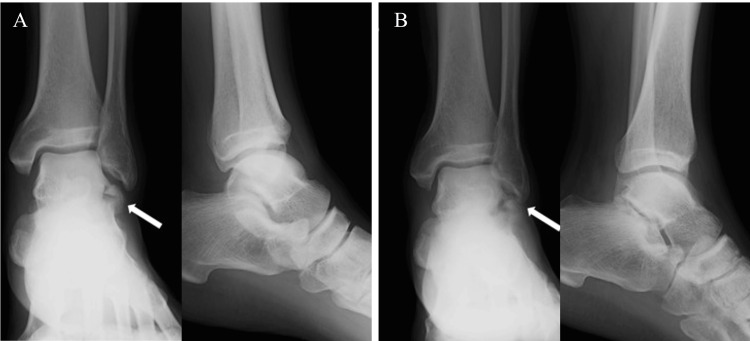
Plain radiograph findings. A: Plain radiographs at the time of injury. Fracture of the lateral process of the talus (FLPT) was observed. B: Plain radiographs at three months after the injury. Dislocation of bone fragments was increased.
